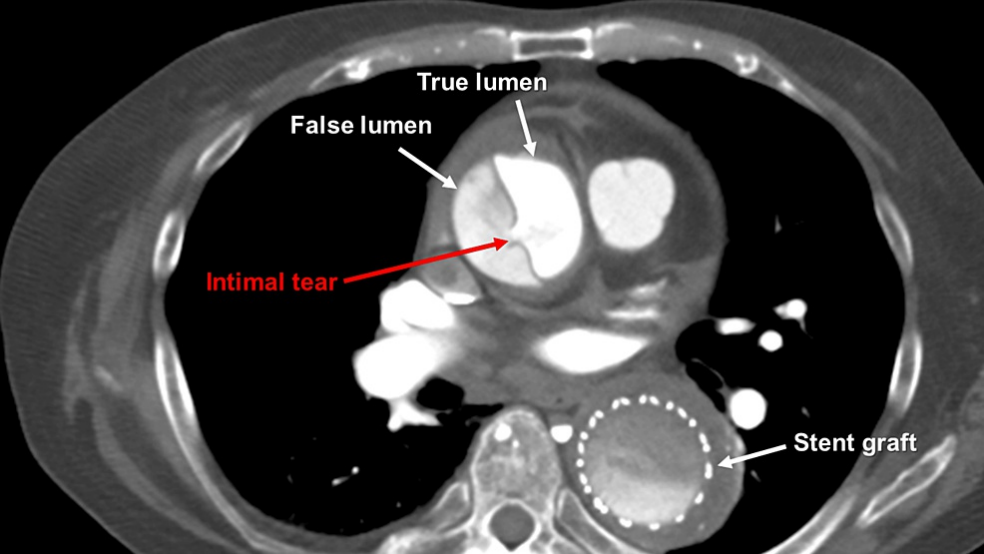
Preoperative computed tomography A preoperative computed tomography scan revealed an intimal tear at the ascending aorta.
